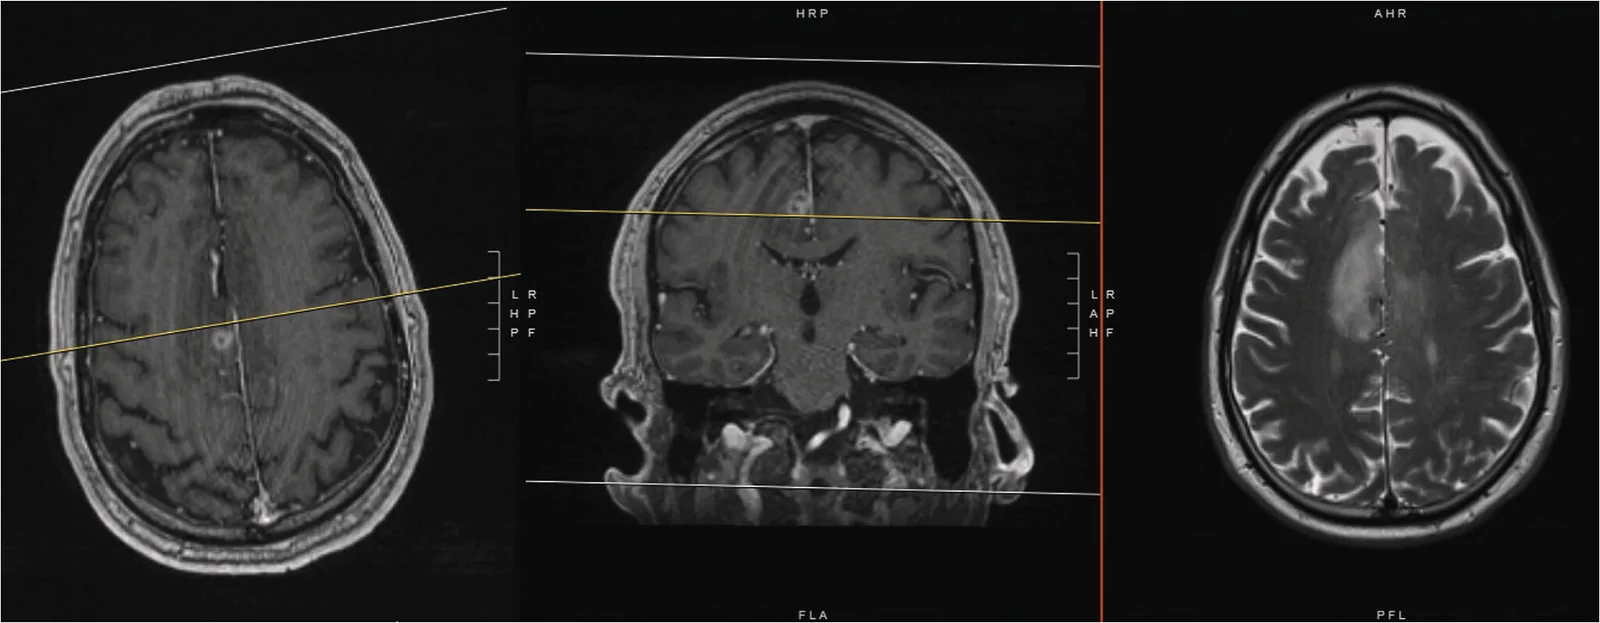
Preoperative MRI showing right frontal glioblastoma (GBM).
